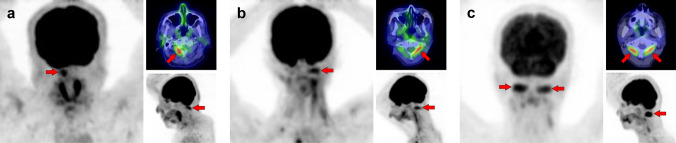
Obliquus capitis inferior muscle.* We can frequently find faint [^18^F]FDG uptake when closely observed, but occasionally experience surprisingly strong uptake in the obliquus capitis inferior muscle. It may be misdiagnosed as a metastatic lesion if it is visualized unilaterally. Therefore, it would be important to understand the pattern of this muscle. Three cases with unilateral uptake (a, b) and bilateral uptake (c) of the obliquus capitis inferior muscle (arrows) are shown
